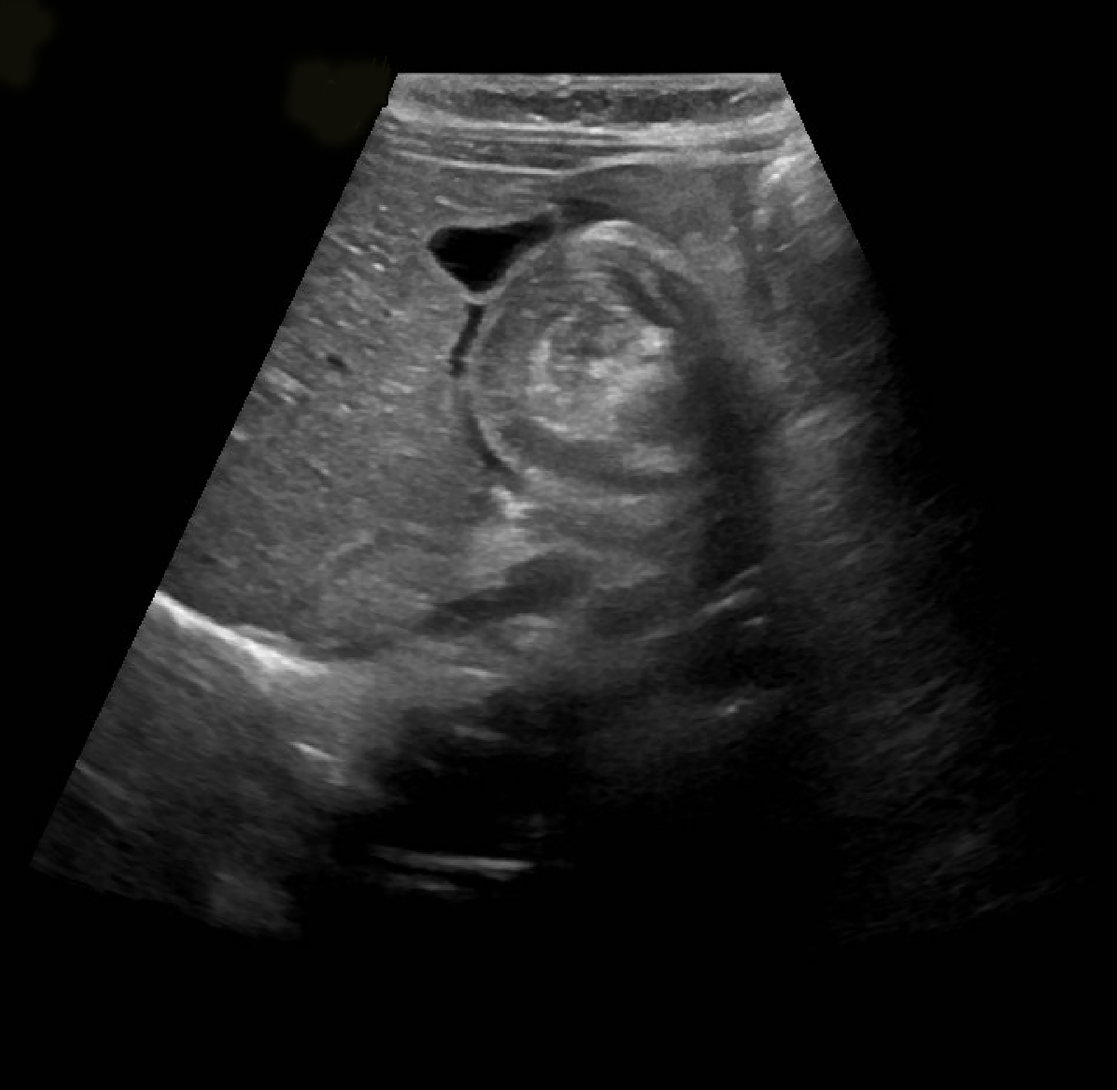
Ultrasound image showing characteristic target sign.
